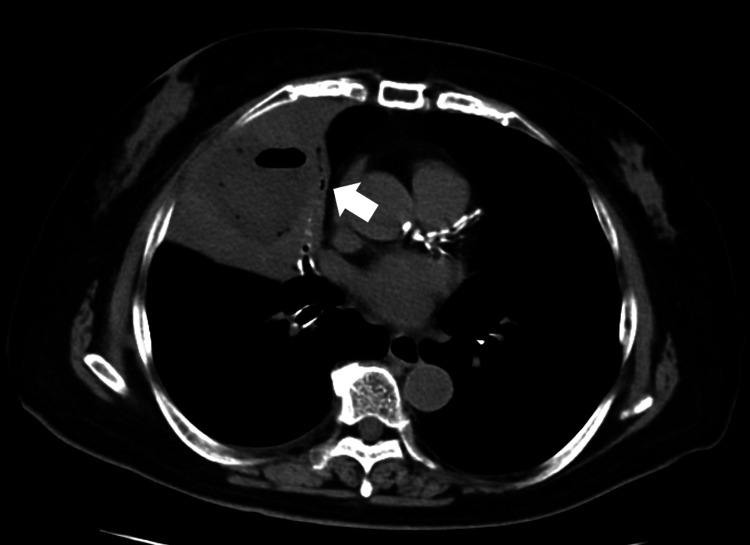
Computed tomography scan performed upon readmission of Case 2 showed the air-fluid level in the right lung proximity of the hepatic resection site (arrow).
